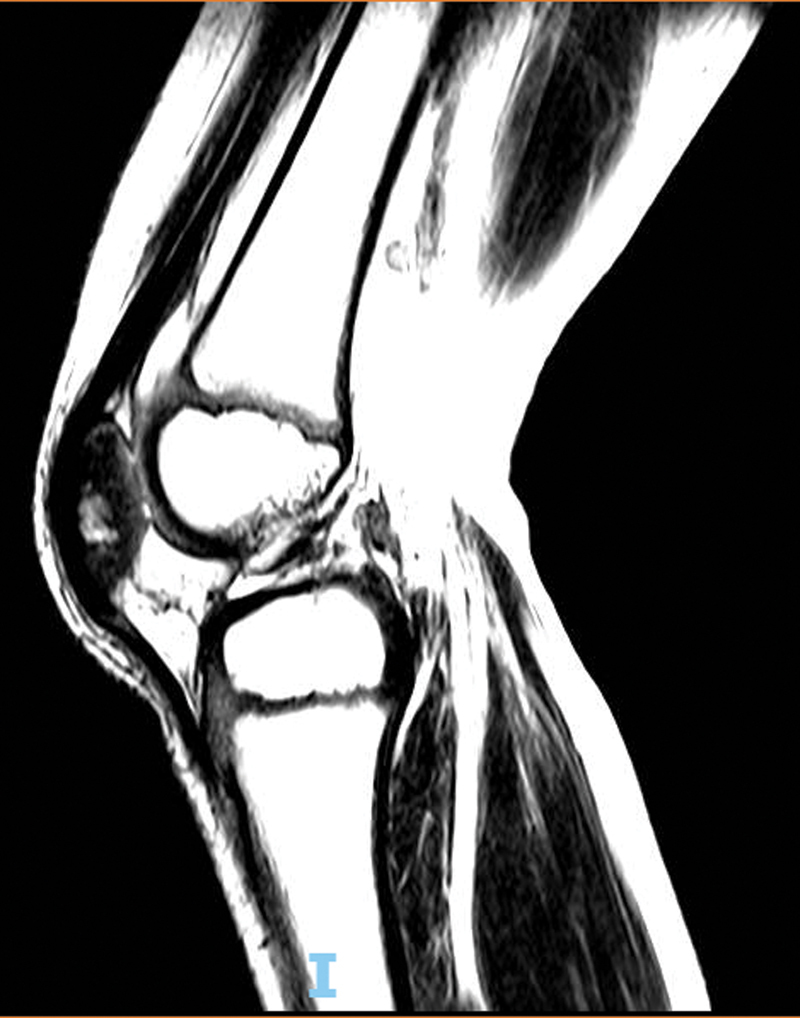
Sagittal, T1-weighted magnetic resonance image of the right knee (August 23, 2021) showing no abnormalities after tuberculosis treatment.
